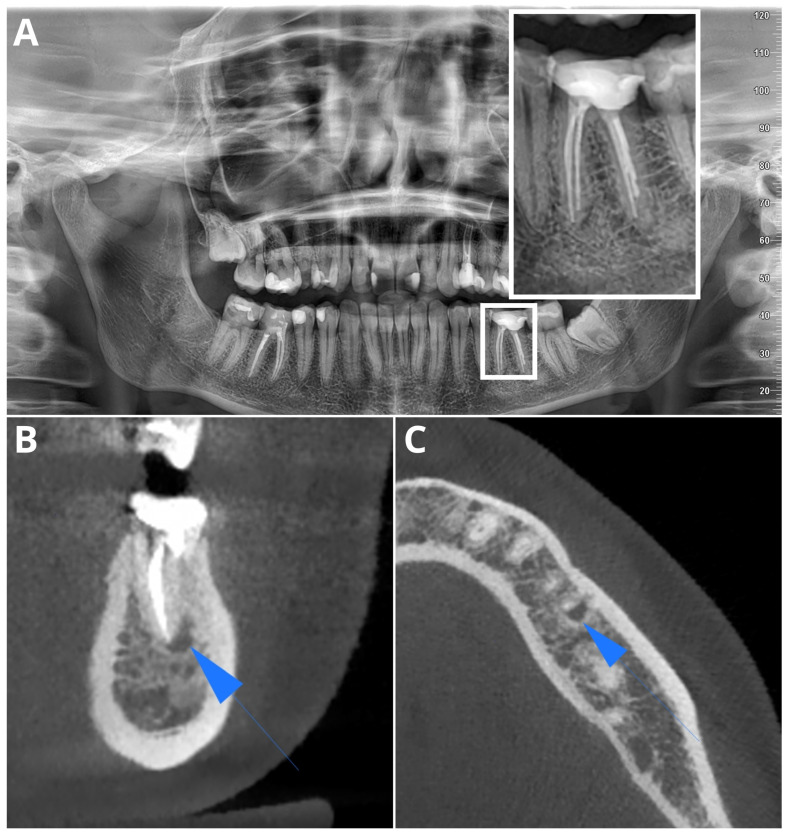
Sample false negative AI diagnosis in OPG, diagnosed by readers in OPG (A) (lesion magnified in white rectangle). PL confirmed in CBCT (blue arrows): coronal plane (B), axial plane (C).
